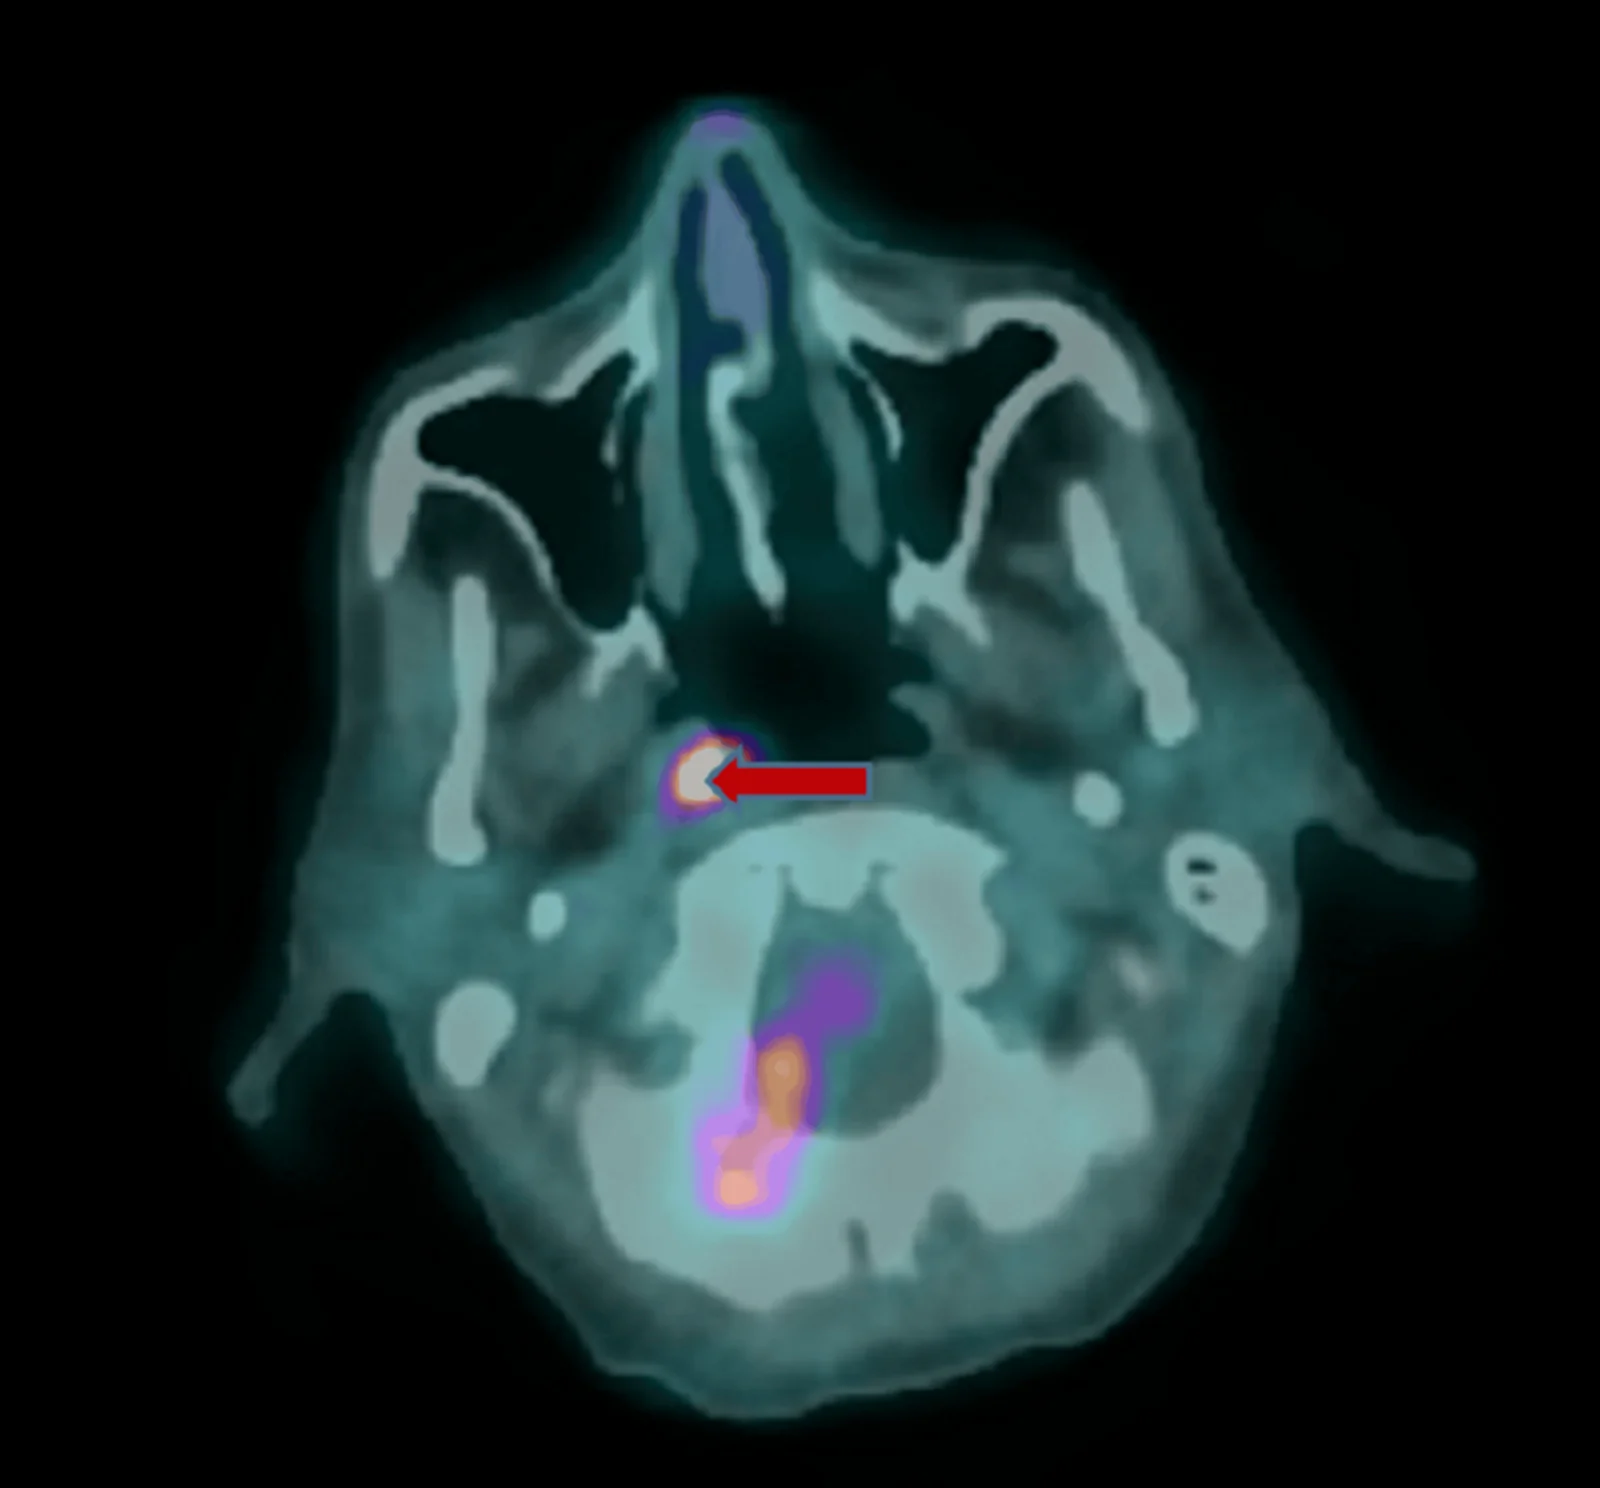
Positron emission tomography computed tomography of the nasopharynx showing good response after induction treatment (arrow)
